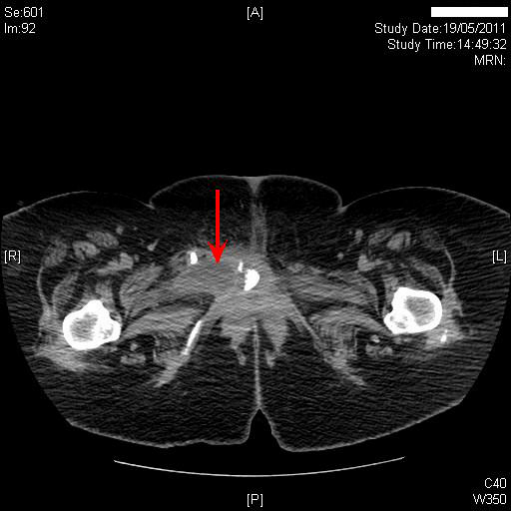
Computed tomography, performed eleven months after first CT, revealed collection of fluid surrounding the right adductor muscle measuring about 2.7 × 4.8 cm in maximum transverse dimensions (red arrow).
